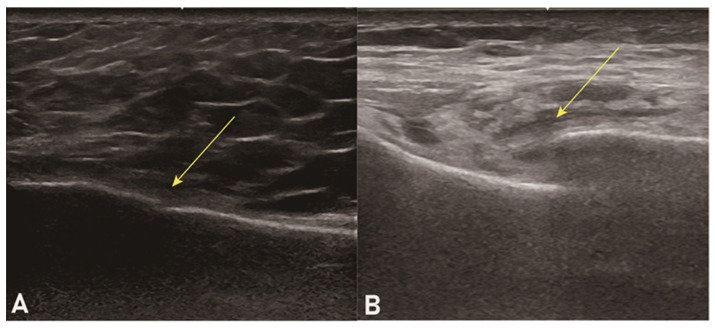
Two example images of sternal ultrasound showing positive findings with loss of continuity in the sternal cortex (arrows) ((A): minimal displacement, (B): moderate displacement).
